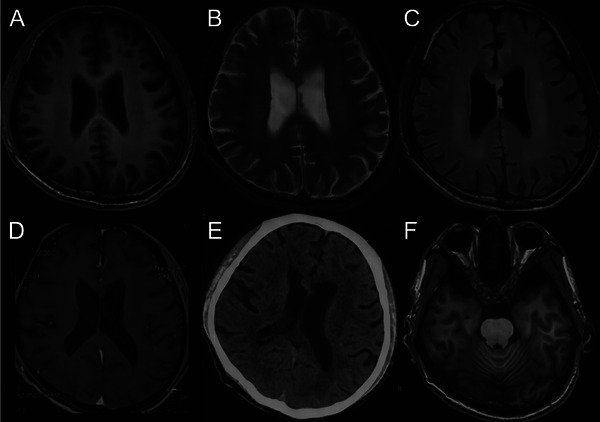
Brain imaging of Patient 1. Multiple subependymal nodules were seen in lateral ventricles and septum pellucidum, predominantly at the roof level (A‐C). The nodules showed no enhancement on gadolinium magnetic resonance imaging (MRI) (D). A computed tomography scan found no calcification (E). Brain MRI also showed periventricular, subcortical, and deep white matter changes with sparing of U‐fibers (C) and temporal poles (not shown). Mild frontotemporal hypoplasia (F), brain atrophy, and ventricular enlargement were noticed.
